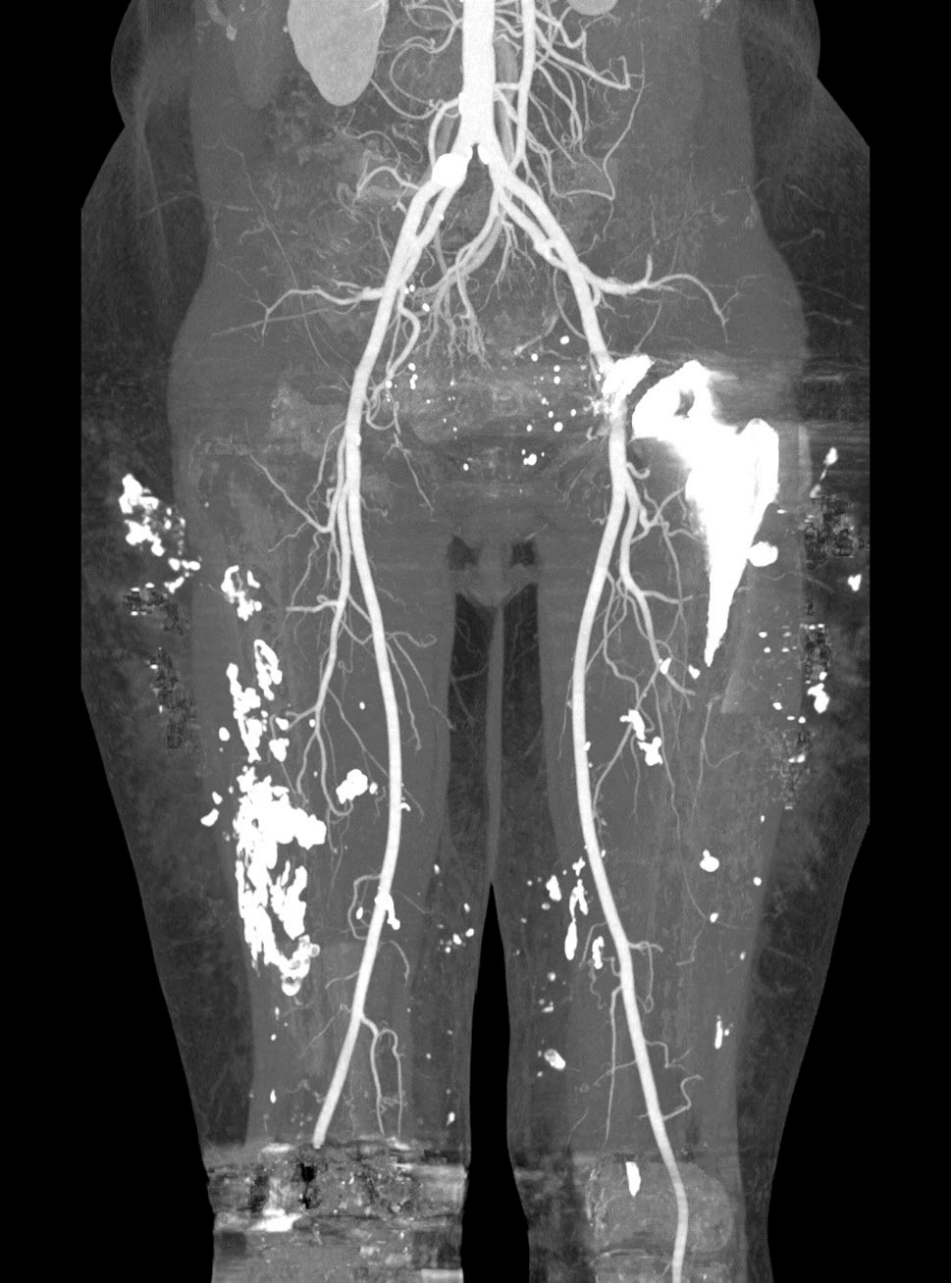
Preoperative CT angiography demonstrating soft tissue calcifications in both thighs
